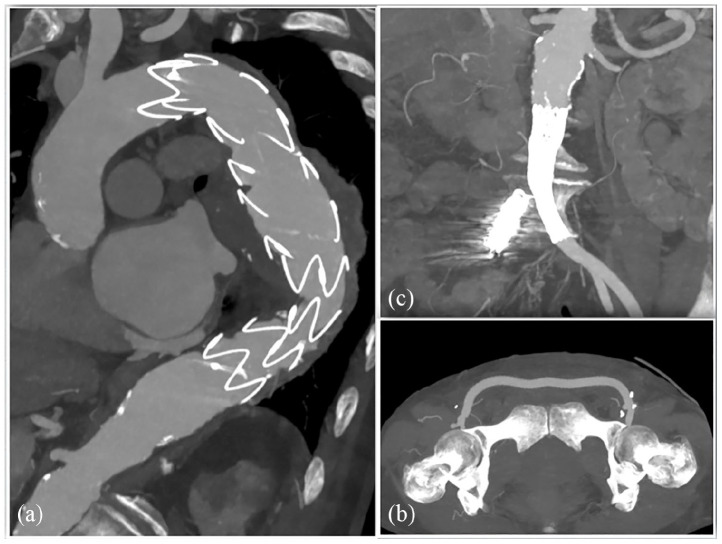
Six months follow-up CTA showing correct aneurysm exclusion (a); regular patency of the stent grafts and bypass (b and c) and no endoleaks.
